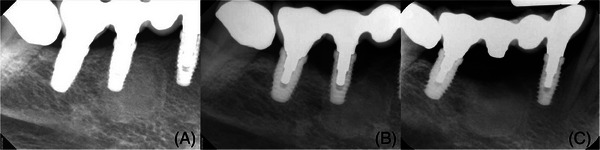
Radiographic series for patient 4. (A) a periapical radiograph taken at the time of prosthesis installation in 2020; (B) in 2022, the middle implant showed advanced horizontal, circumferential crestal bone loss and was removed and the outcome appeared stable one year later (C).
